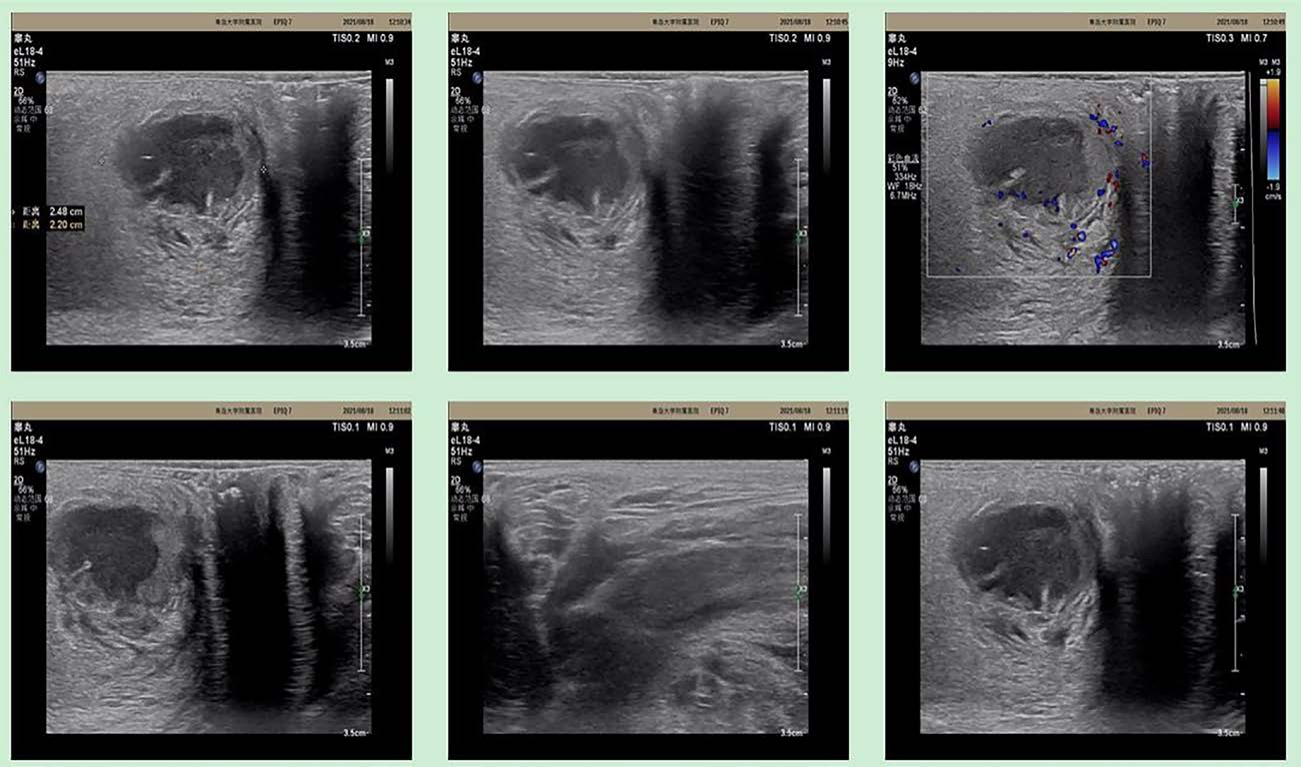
Patient’s perianal ultrasonographic findings. Perianal 2.5 × 2.2 cm mixed echogenic mass between 12 o’clock position, morphology is still regular, border is still clear, liquid dark area is seen inside, poor translucence, flow sensation can be seen by probe pressure, and clear demarcation with anal canal, consider perianal abscess.
